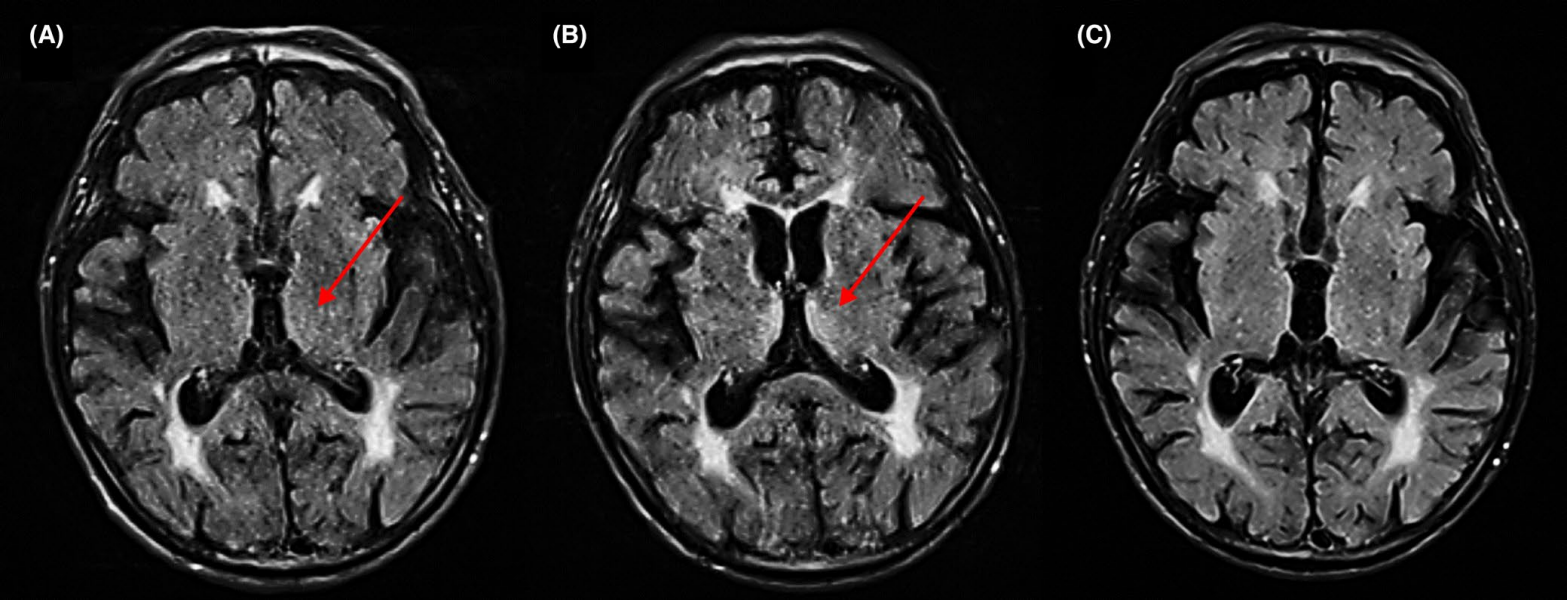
Findings of head magnetic resonance imaging with T2‐weighted fluid‐attenuated inversion recovery sequences (A) on admission with loss of appetite, (B) on the 14th day of admission with Wernicke's encephalopathy, and (C) at 1 month after thiamine injection. Red arrows indicate symmetric increased signals inside the thalami, a common and characteristic finding of Wernicke's encephalopathy
